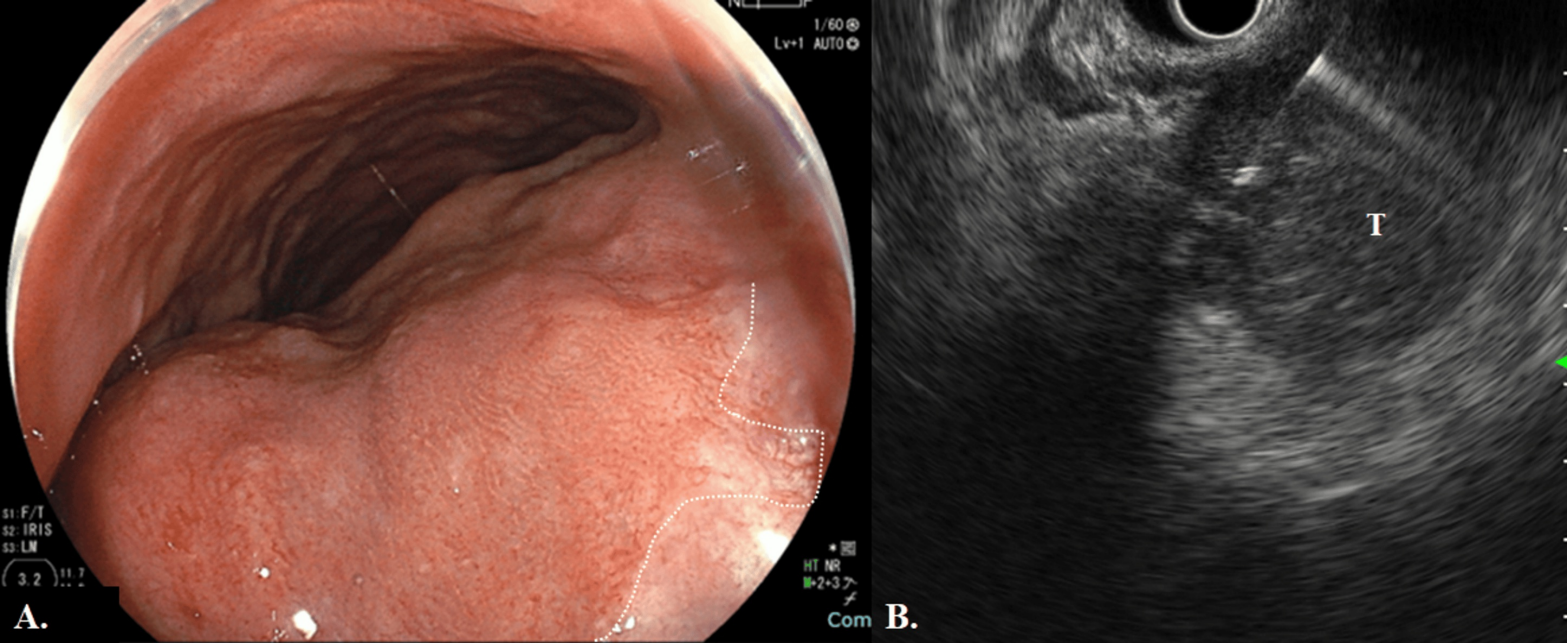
Colonoscopy and endoscopic ultrasound images (A) Pretreatment colonoscopy showed extramural compression of the lower rectum (Rb). The distance of the lesion from the anal verge was about 3 cm, and the lower margin of the lesion was located as high as the dentate line (white dotted line). (B) Endoscopic ultrasound-guided fine needle aspiration was performed at another hospital. T, tumor
